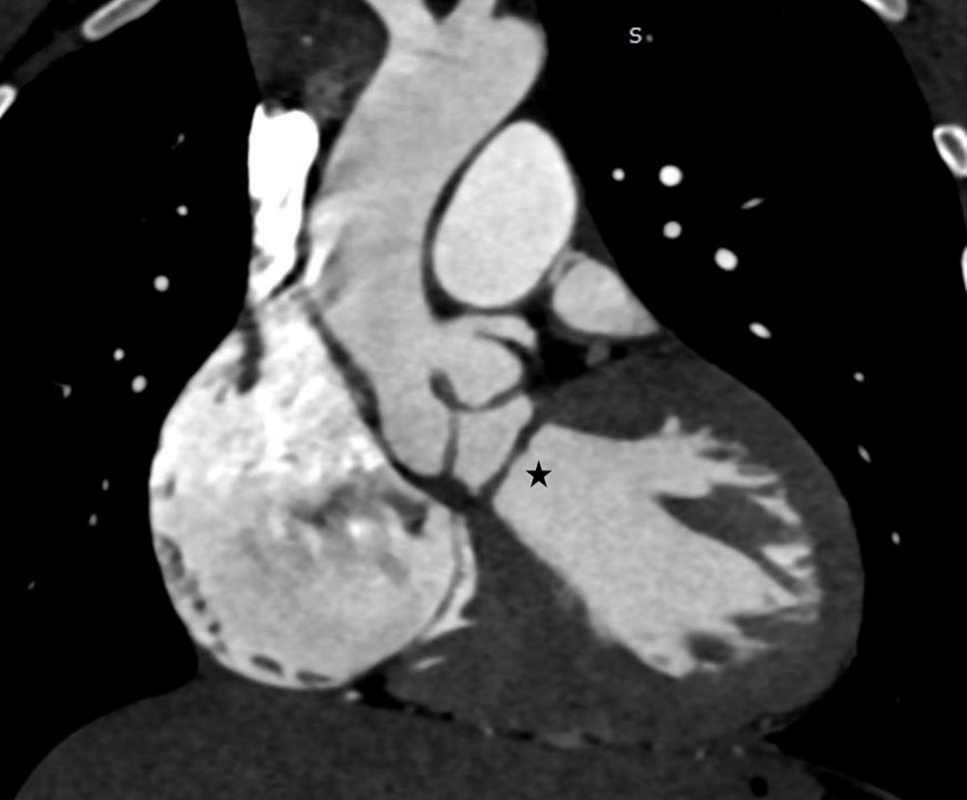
Cardiac CT showing subaortic membrane in the left ventricular outflow tract (star) beneath the aortic annulus
